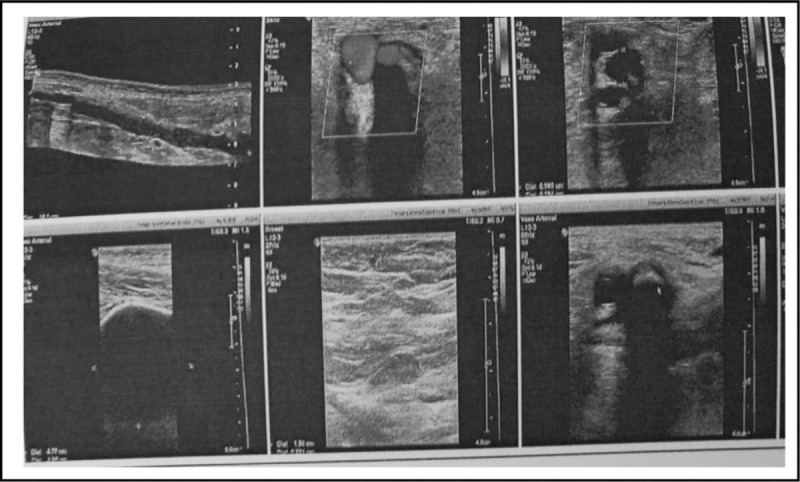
Postoperative color-Doppler ultrasound images of vessels. Bone cement presents along the intima of femoral and iliac vein wall, which causes luminal stenosis, but blood flow is fluent.
